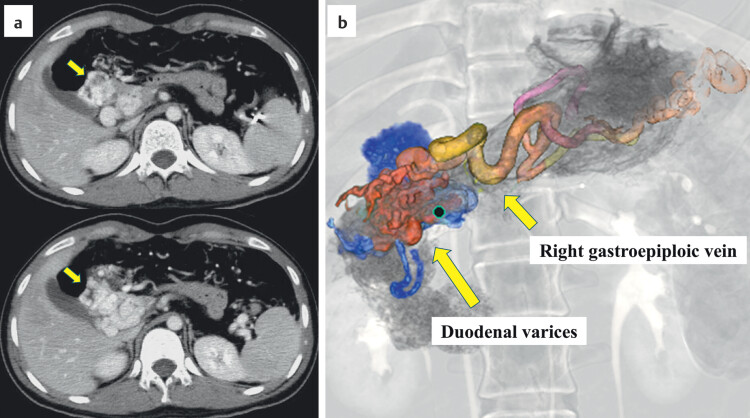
Contrast-enhanced computed tomography (CT) and 3D-CT findings.
